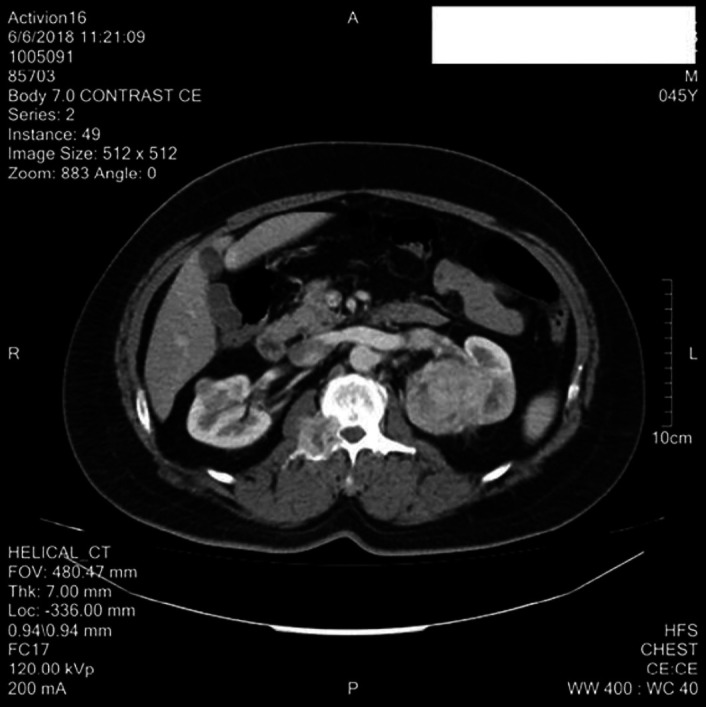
CT scan showing left renal mass along with the destruction of the right pedicle of the D12 vertebra
